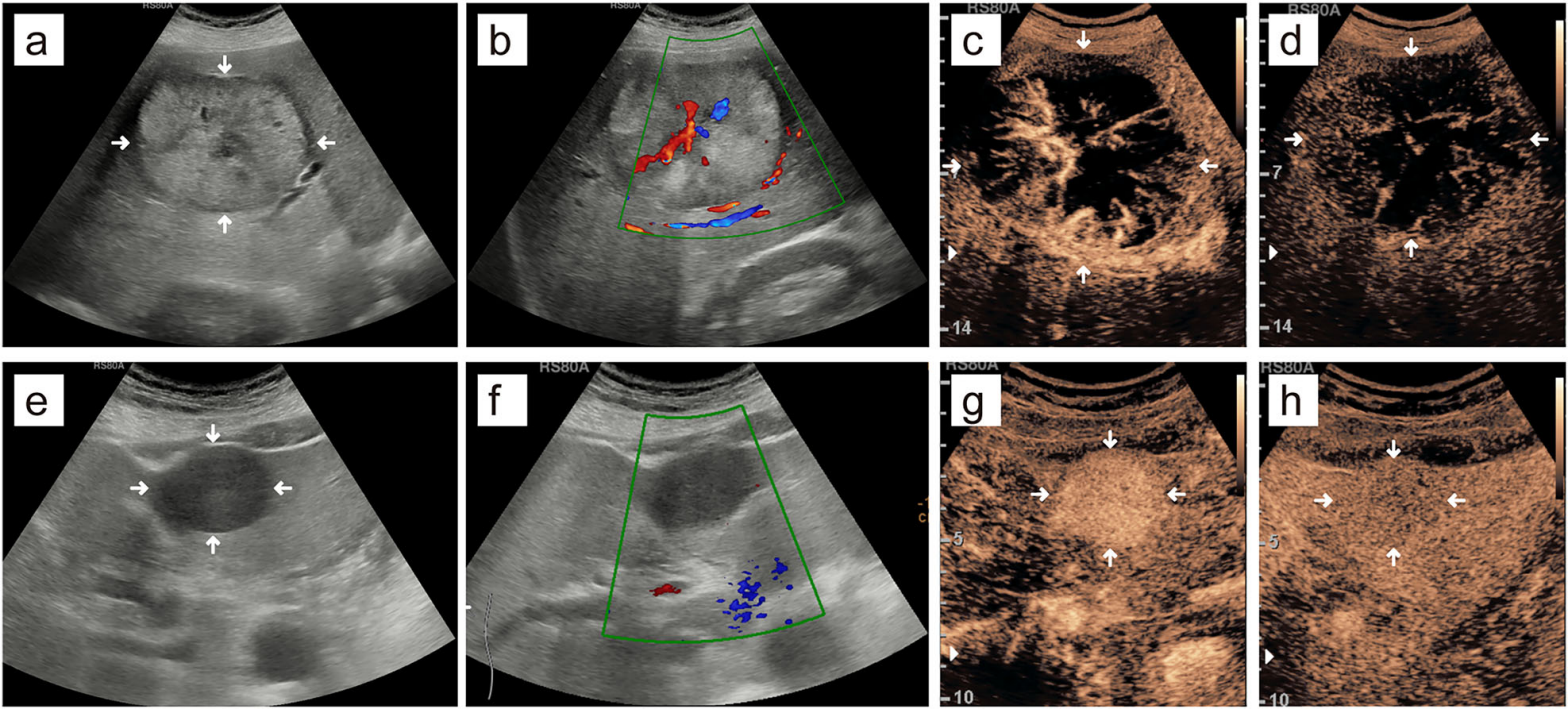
a–d Typical US/CEUS images in a 50-year-old man with hepatitis B virus-related HCC, positive for both MTM-HCC subtype and high Ki-67 pattern. a B-mode US showed a 10.3 cm isoechoic HCC lesion with halo sign located in the right liver lobe. b CDFI showed the intratumoral artery and peritumoral nutrient vessel. c Arterial phase branch-like heterogeneous hyperenhancement with a large area of nonenhancement was observed at 20 s after contrast agent injection. d Portal vein phase marked hypo-enhancement was observed at 101 s after contrast agent injection. e–h Typical US/CEUS images in a 72-year-old man with autoimmune hepatitis-related HCC, negative for both MTM-HCC subtype and high Ki-67 pattern. e B-mode US showed a 4.0 cm hypoechoic HCC lesion located in the left liver lobe. f CDFI showed no significant colored blood flow. g Arterial phase homogeneous hyperenhancement was observed at 20 s after contrast agent injection. h Portal vein phase iso-enhancement was observed at 100 s after contrast agent injection. US, ultrasound; CEUS, contrast-enhanced ultrasound; HCC, hepatocellular carcinoma; MTM, macrotrabecular-massive; CDFI, color Doppler flow imaging
